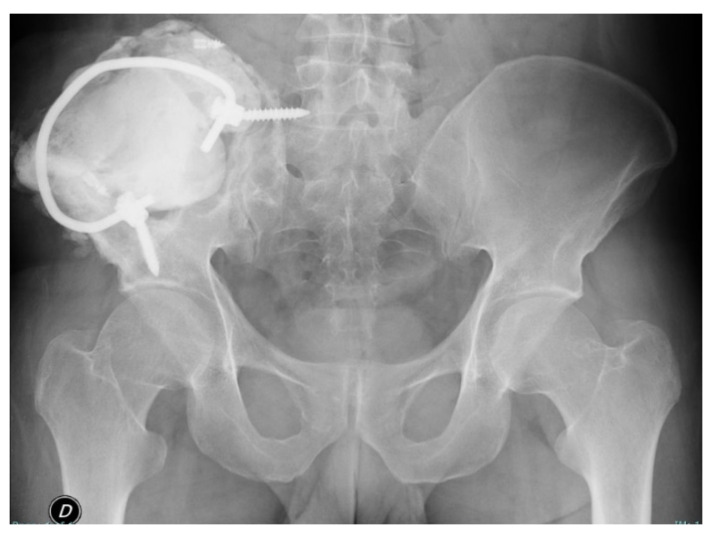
One-month post-operative radiograph control.
